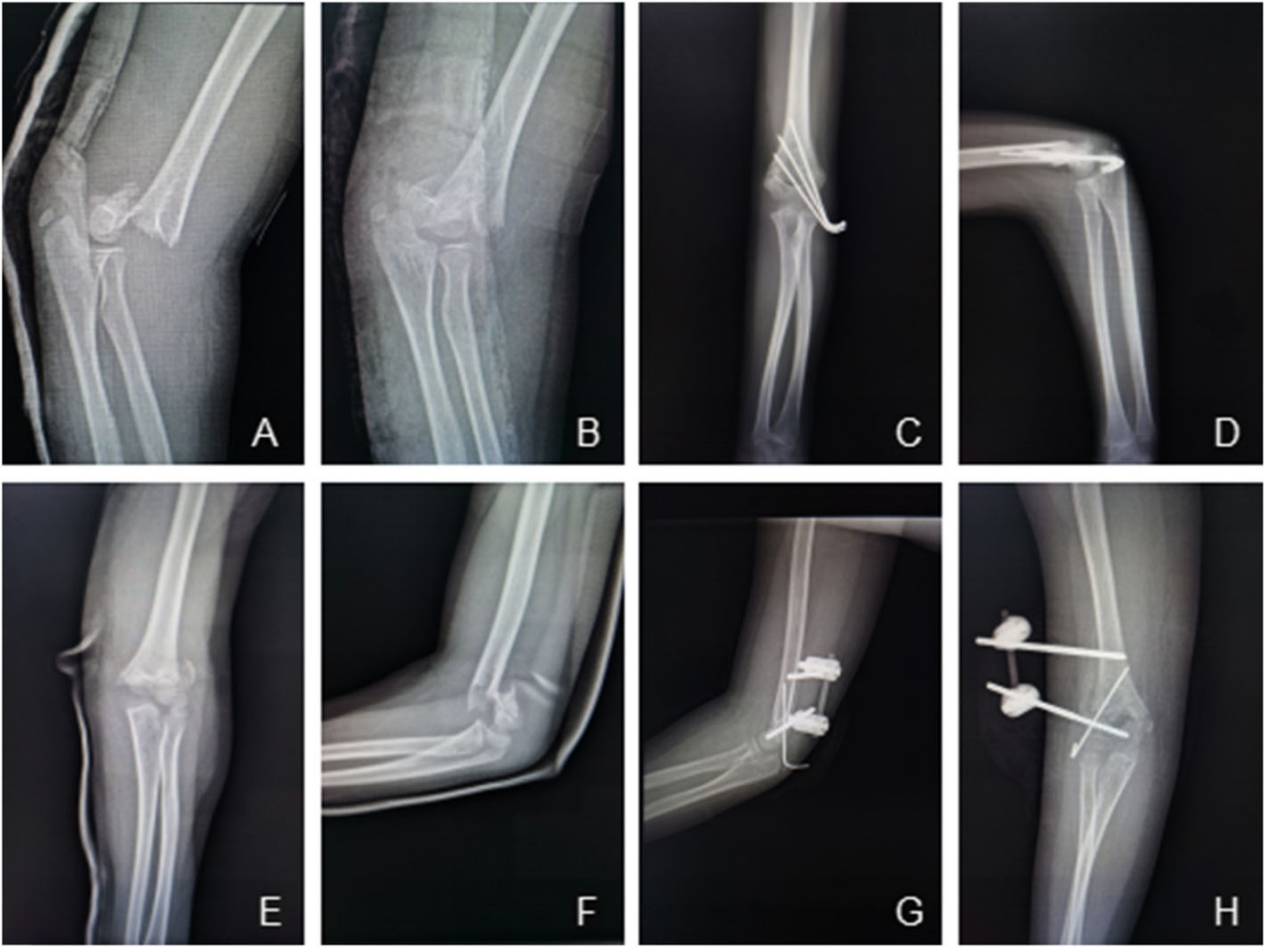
A, B, E, F Two cases of extension-type supracondylar fracture of the humerus in older children. C, D X-ray of the elbow joint after internal fixation with Kirschner wire. G, H X-ray of elbow joint after external fixation
